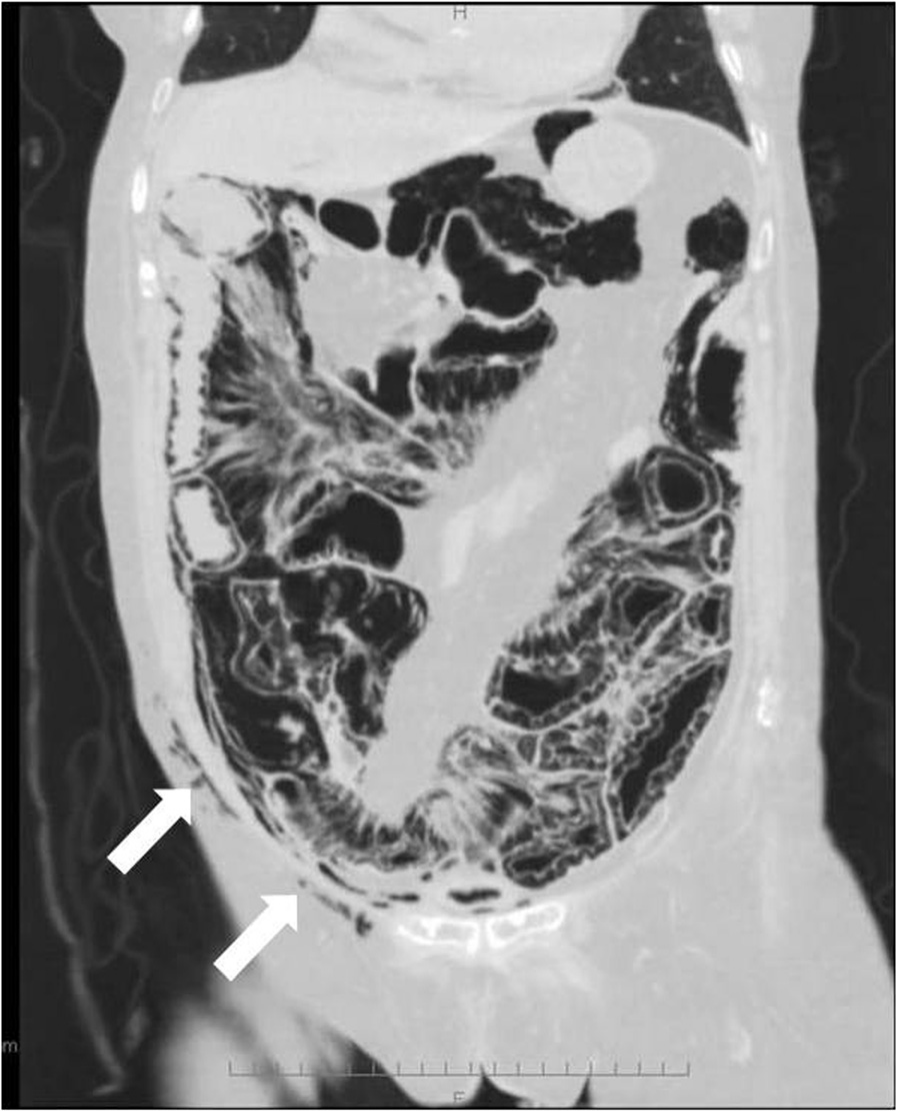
A coronal CT scan of the abdomen showed severe PI and subcutaneous emphysema (*white arrow*). Gas tracking wall is visualized parallel to the intestinal mucosa
